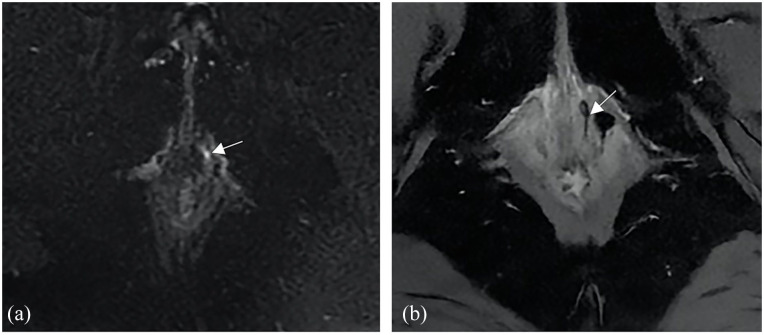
(a) Axial T2-weighted fat-suppressed image shows an intersphincteric fistula with fluid content in the left anterolateral wall of the anal canal (arrow). (b) Axial T2-weighted fat-suppressed image shows the resolution of the fluid content in the fistula (arrow) after the surgical procedure.
